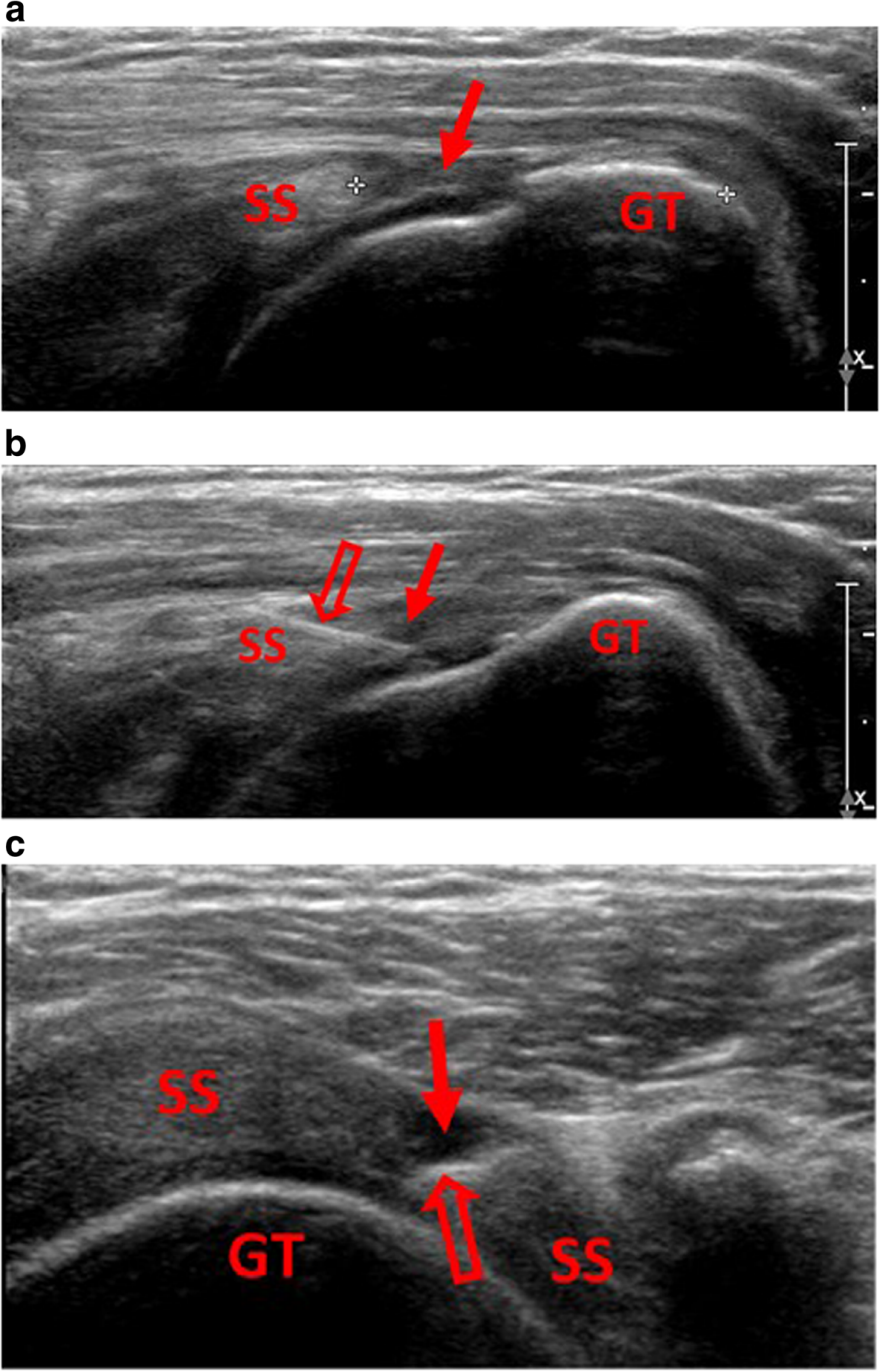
a Rupture size in longitudinal axis of supraspinatus tendon was measured by sonography. Hypoechoic area (solid arrow) indicates the rupture area of tendon. 1b Intra-substance injection was done by echo-guided injection directly into the rupture area (solid arrow) of supraspinatus tendon. Injection needle (hollow arrow) can be clearly seen on sonography in longitudinal view. 1c Injection needle tip (hollow arrow) can be seen in rupture area (solid arrow) of supraspinatus tendon in transverse view SS = supraspinatus tendon, GT = greater tuberosity of humerus.
